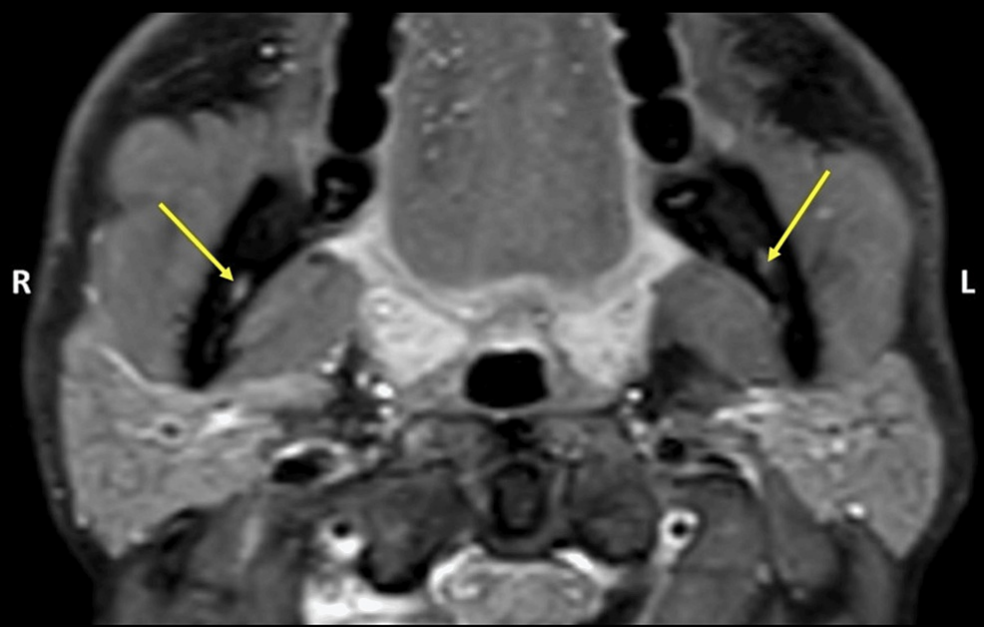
T1-weighted axial section showing the entry of the inferior alveolar nerve in the mandibular canal (yellow arrows)
